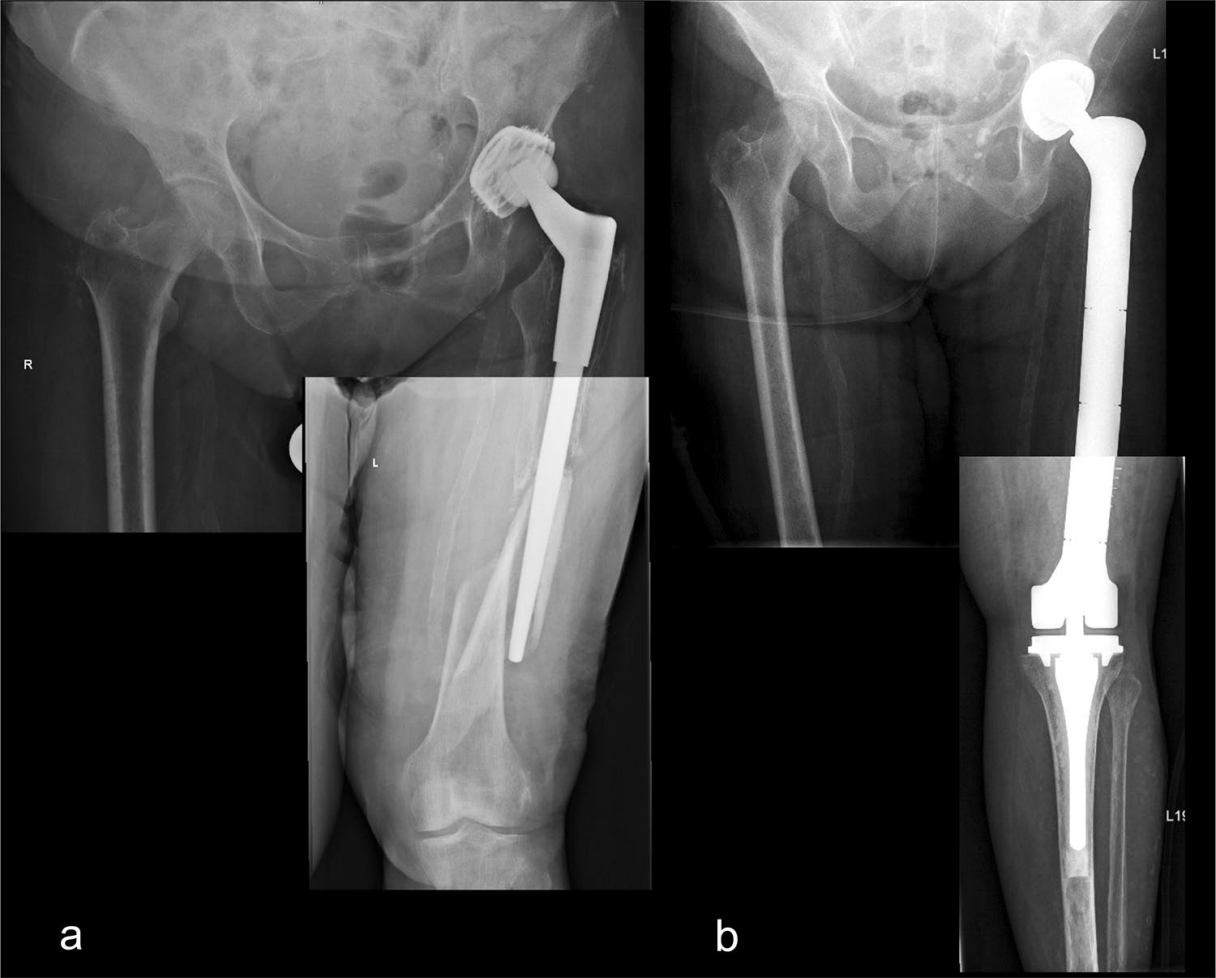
Preoperative (a) and postoperative x-rays (b) of a total femoral replacement after recurrent periprosthetic fracture following a total hip replacement in a female patient with multiple comorbidities. A swift performance of surgery was necessary.
